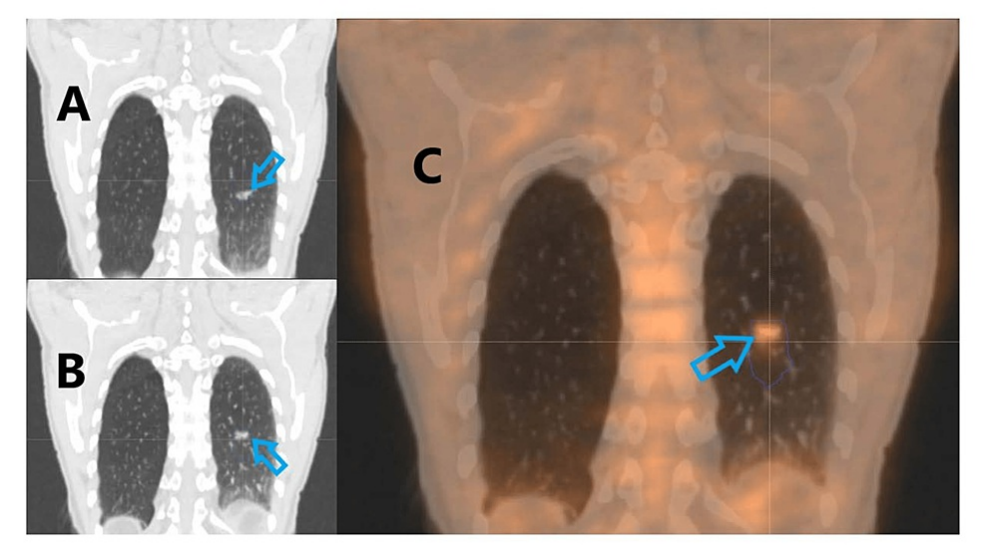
Lung tumor realized by 4DCT showing superior and inferior extremes of motion (A) Inferior extremes of motion (B) Superior extremes of motion (C) Demonstration of PET/CT's potential not only for tumor identification, but also for tumor real-time tracking. 4DCT: Four-dimensional computed tomography, PET/CT: positron emission tomography/ computed tomography This image is permitted through the signed consent from respective University of Mississippi Medical Center patient
